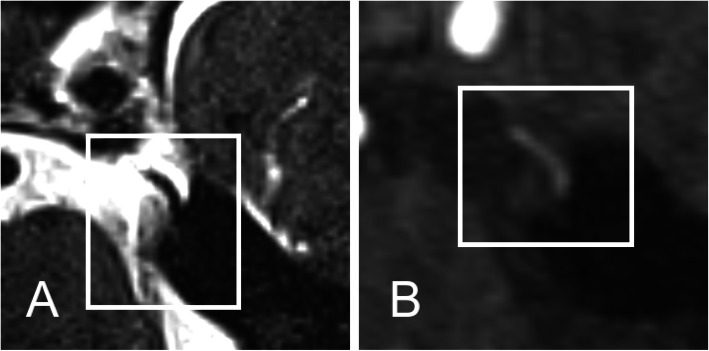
a NVC of SCA and CN V: (A) MRI-SPACE (B) MRI-TOF. A: MRI-SPACE sequence with neurovascular compression of the trigeminal root entry zone by a loop of the superior cerebellar artery. Note the highly-resoluted T2 enhanced sequence with hyperintense cerebrospinal fluid space containing hypointense vessels and cranial nerves. b MRI-TOF (time of flight angiography) showing the hyperintense arterial flow within the superior cerebellar artery.
